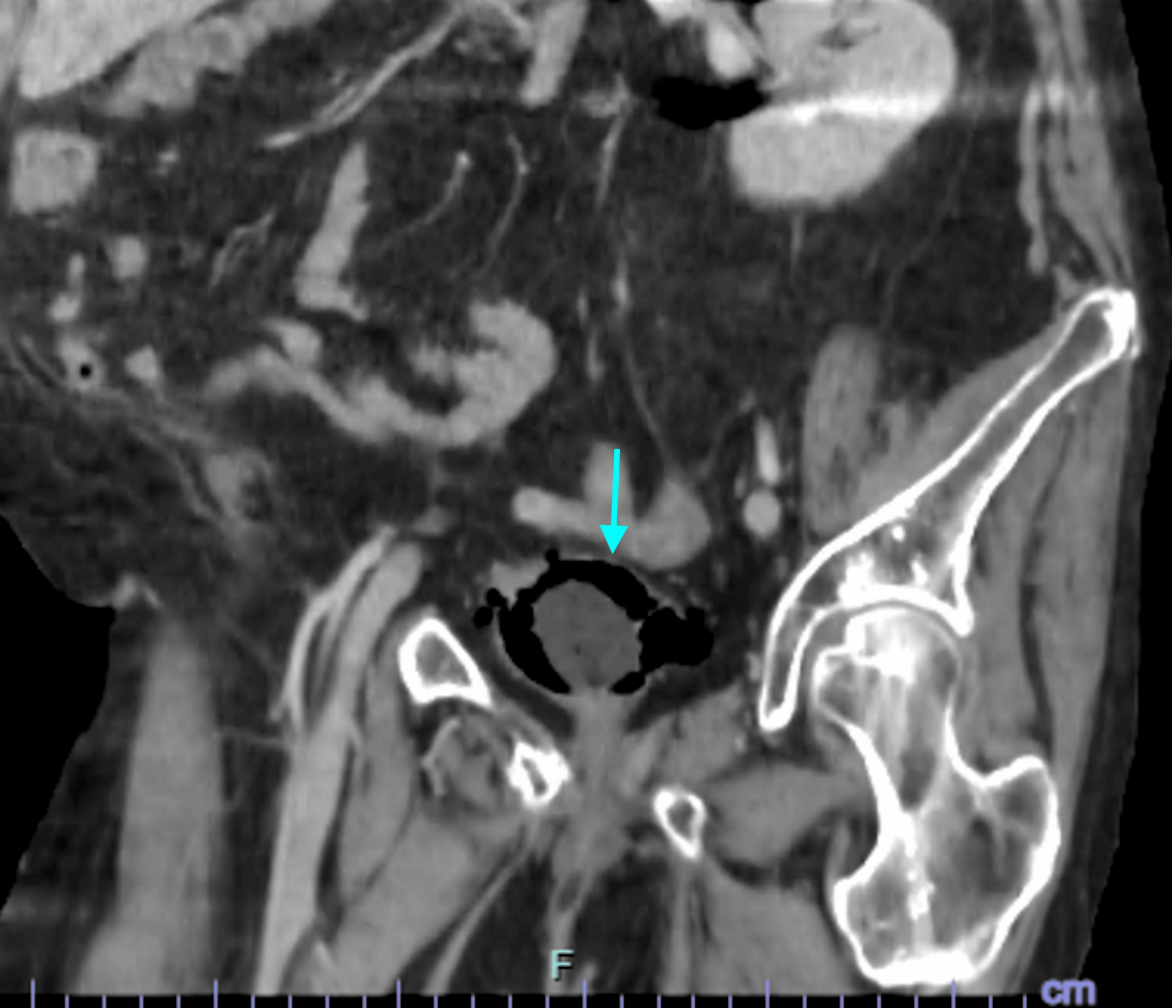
Coronal computed tomography reconstruction demonstrating craniocaudal extension of gas into the retropubic and extraperitoneal pelvic spaces adjacent to the urinary bladder
